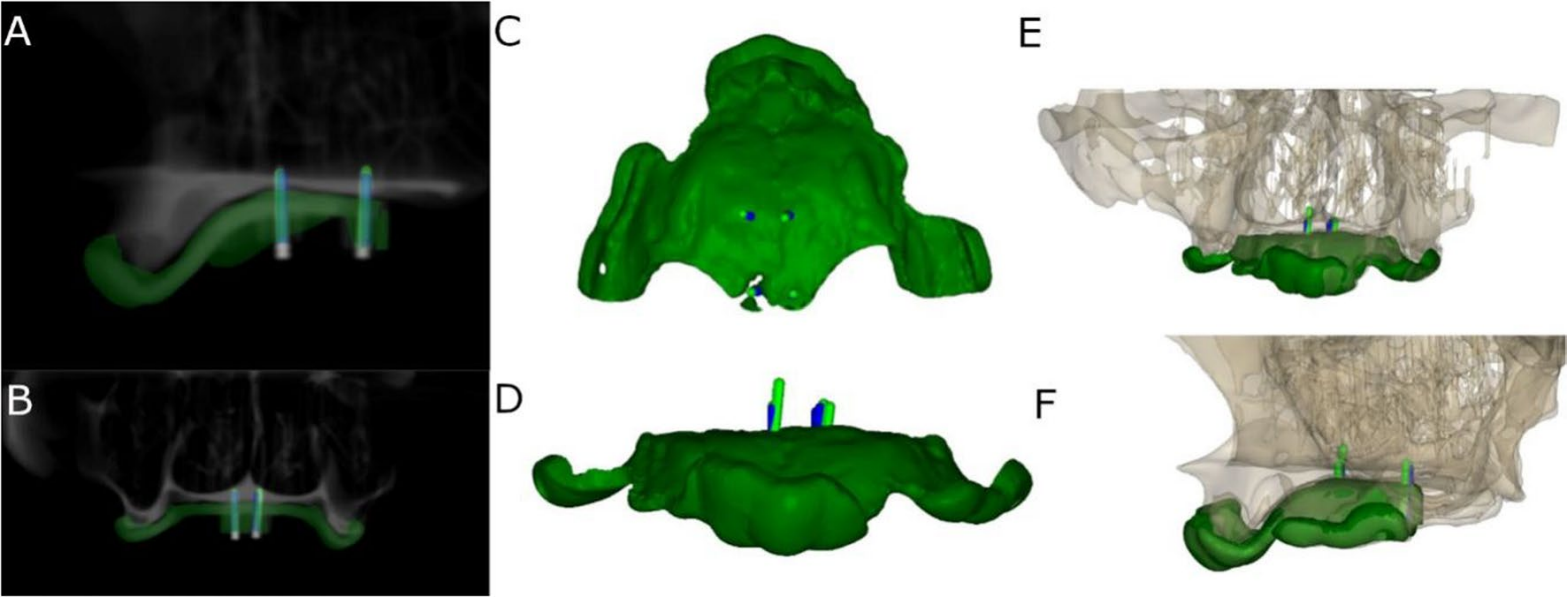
A Sagittal and B coronal view of the virtually planned (blue cylinders) and performed orthodontic self-drilling mini-implants (green cylinders) and surgical template (green line) on the CBCT scan, C top and D front view of the virtually planned (blue cylinders) and performed orthodontic self-drilling mini-implants (green cylinders) and surgical template and E front view and F sagittal view of the virtually planned (blue cylinders) and performed orthodontic self-drilling mini-implants (green cylinders) and surgical template in the completely edentulous upper maxilla
